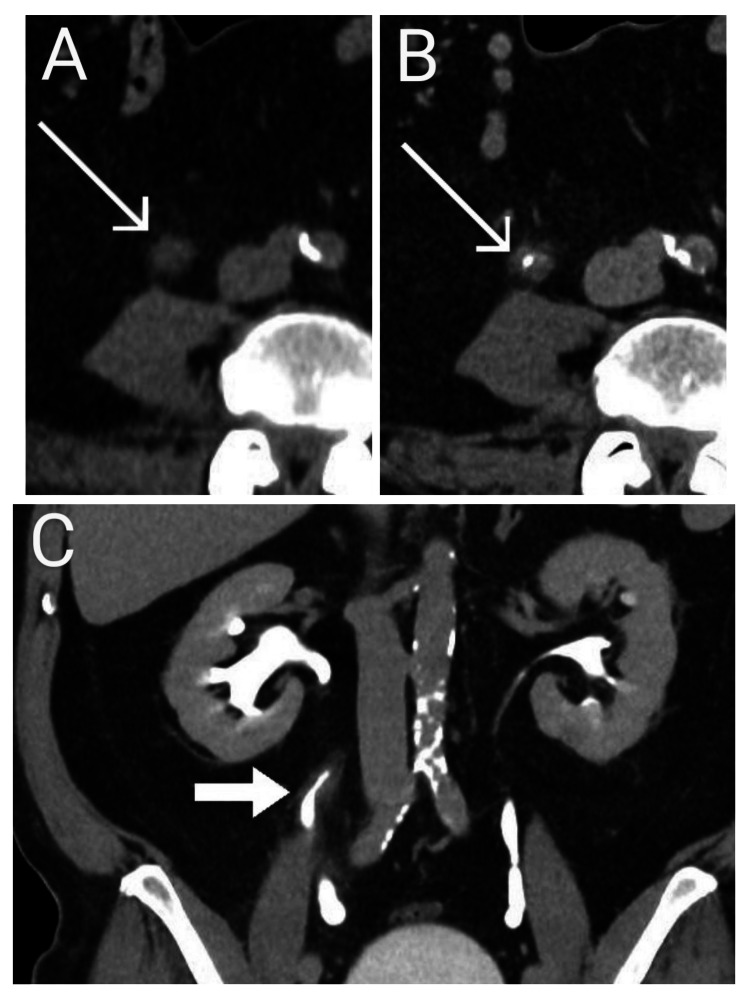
Standard venous phase (A), along with axial (B) and coronal (C) views in the excretory phase. CT showed diffuse thickening of the wall in the right mid-ureter, along with a region of relatively constricted lumen in the mid-ureter. There was no discrete mass or other collecting system filling defects detected.
